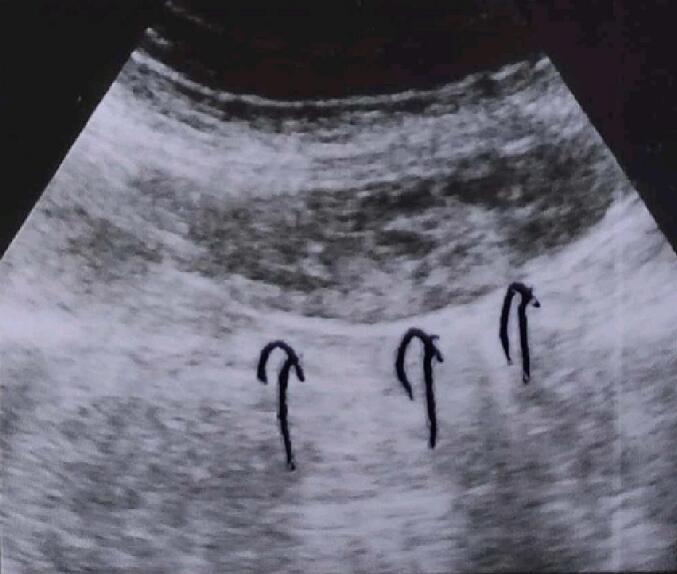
Ultrasound of the abdomen and pelvis, Cystic formation in rectus abdominis.
